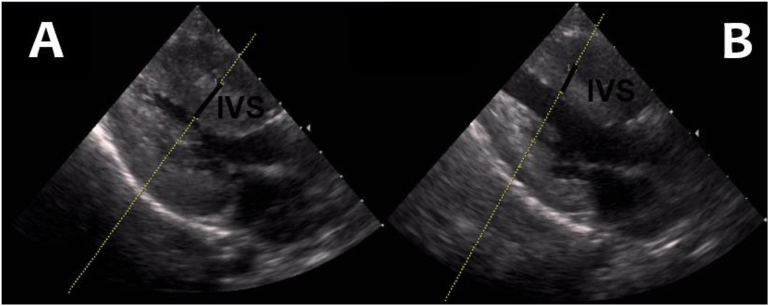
Echocardiographic findings in a patient with glycogen storage disease type III upon detection of hypertrophic cardiomyopathy before therapy (A) and 18 months after the introduction of a high-fat and low-carbohydrate diet (B); *IVS,* interventricular septum.
